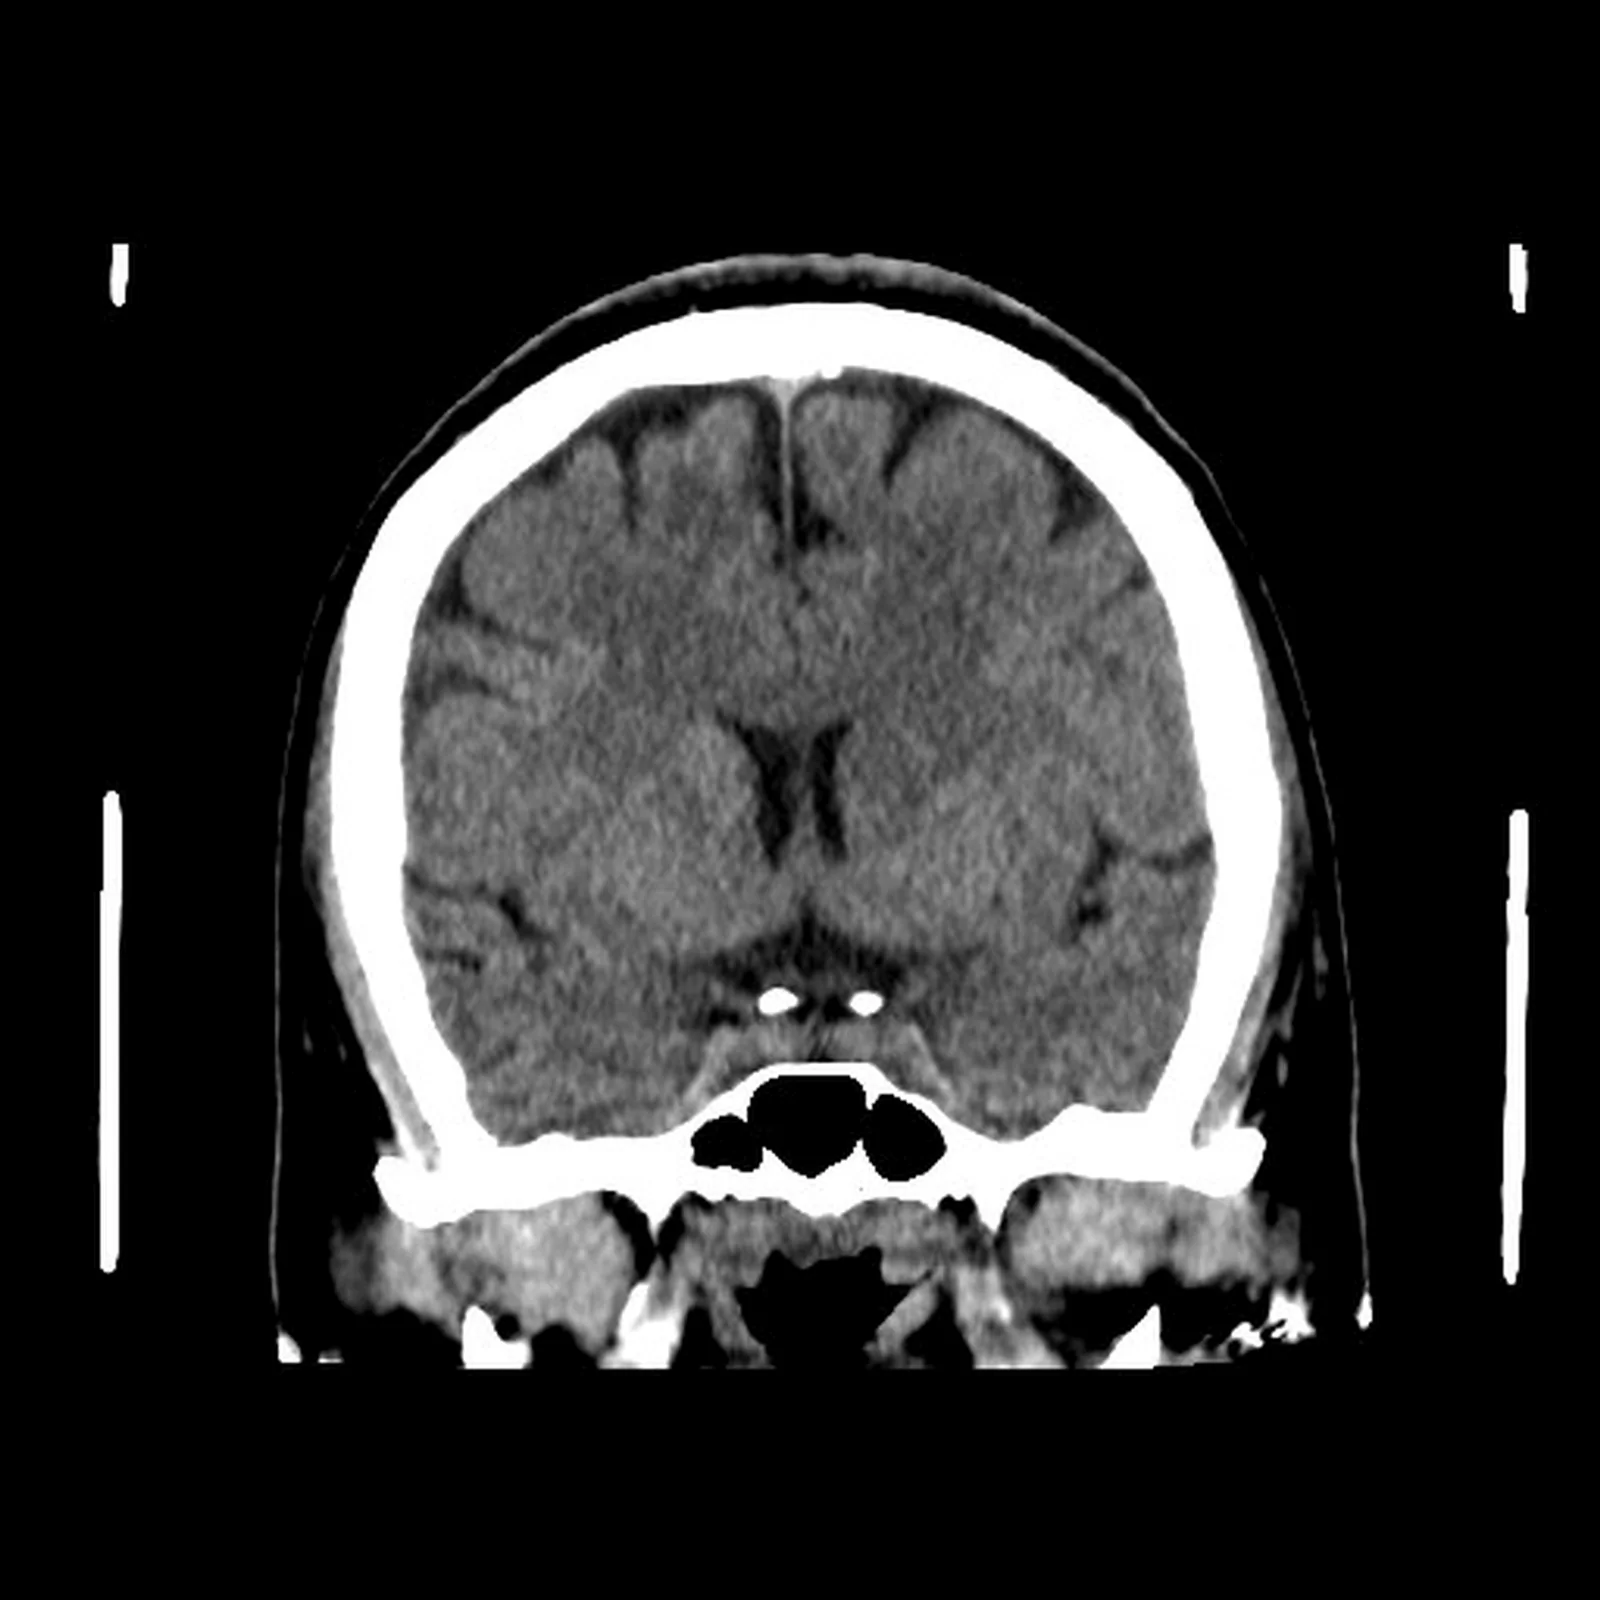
Coronal CT showing bilateral parieto-occipital hypoattenuation. CT: computed tomography.
